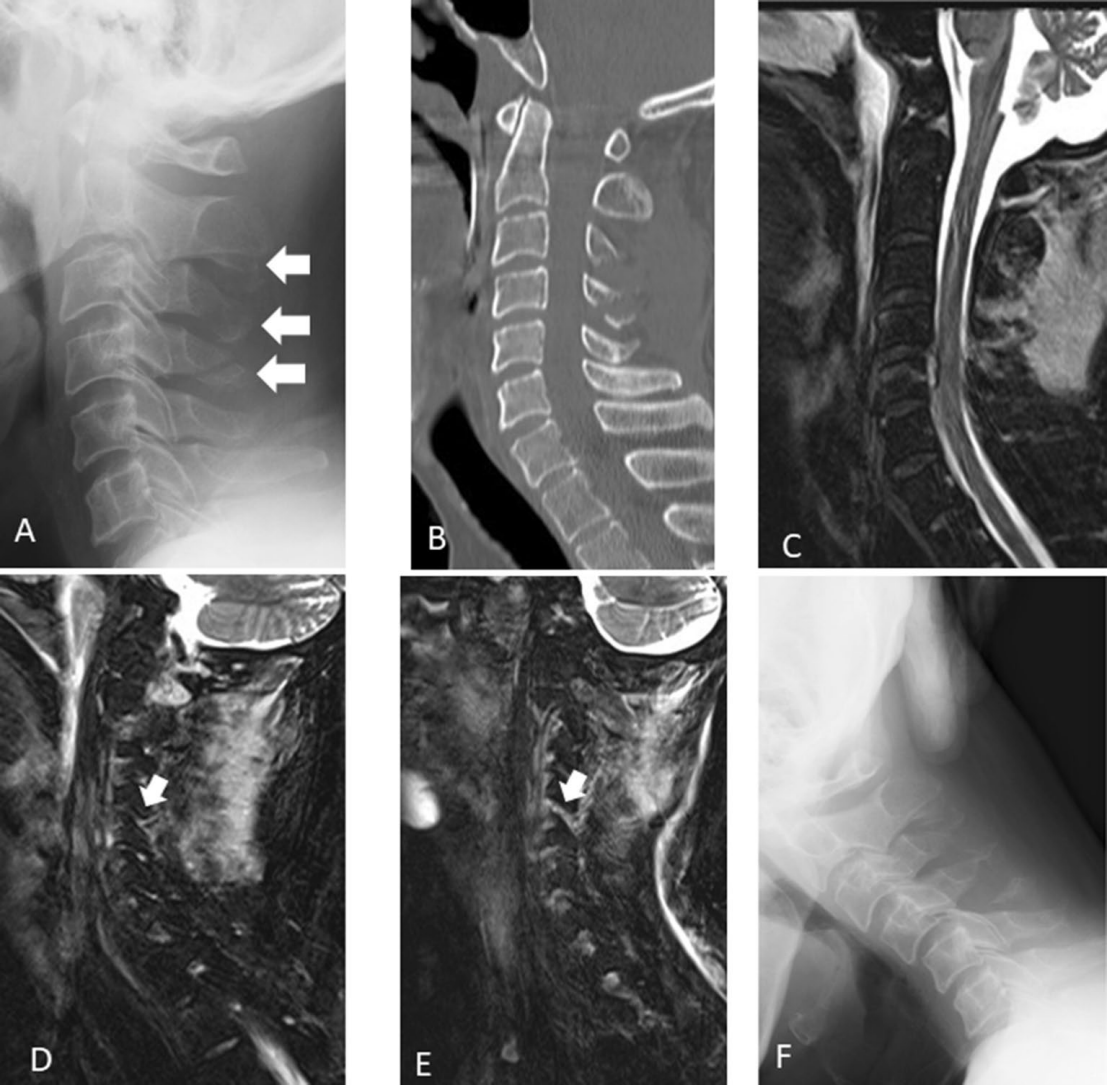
Assessment of cervical instability in a 56-year-old patient. (A,B) Lateral radiography image obtained at neutral position during the initial visit and mid-sagittal view of computed tomography scan. Radiography showed C2, 3, 4 spinous process fracture (white arrow). (C) Mid-sagittal view on magnetic resonance imaging and short tau inversion recovery image. (D,E) C4/5 facet effusion (white arrow). (F) Dynamic radiography showing segmental instability at C4/5.
